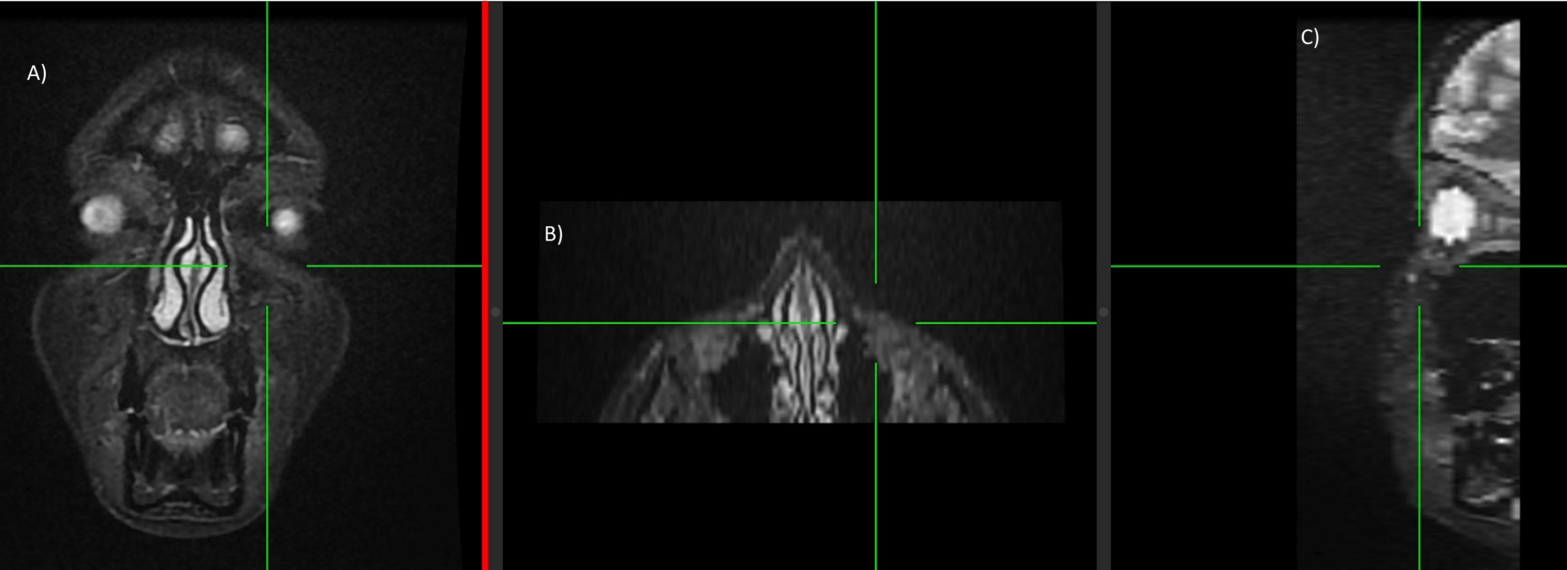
Facial MRI maximum intensity projection (MIP); these images are able to demonstrate in the three spatial planes through a coronal (A), axial (B) and sagittal (C) view in MRI that Juvelook did not alter the signal intensity or radiological pattern in the MRI of the tissues after having been applied (two weeks post injection).
